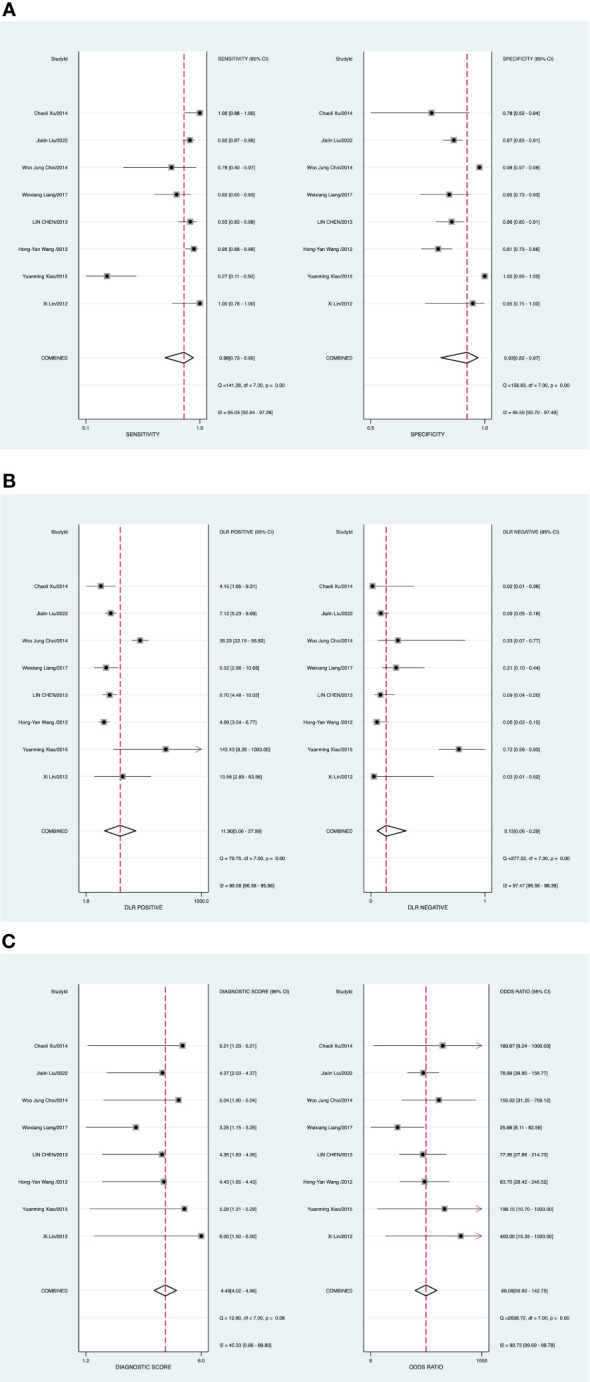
(A) Forest plot of sensitivity and specificity of automated breast ultrasound (ABUS) in the diagnosis of breast cancer. (B) Forest plot of the diagnosis likelihood ratio (DLR). (C) Forest plot of the diagnostic odds ratio (DOR).
